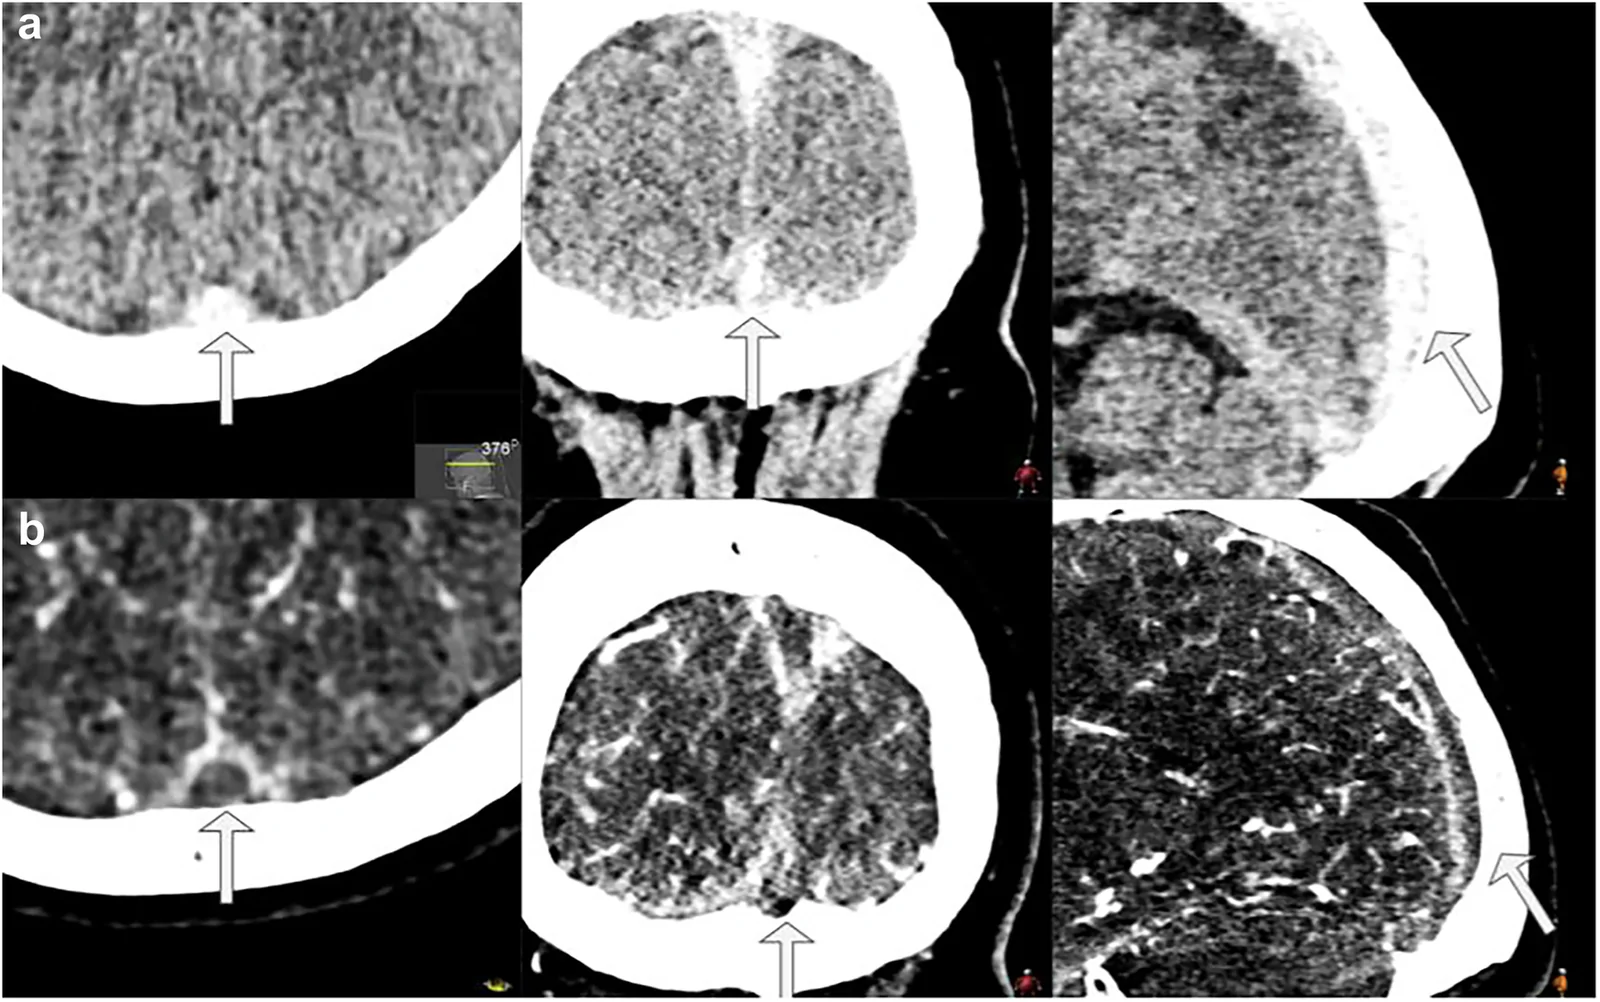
Acute superior sagittal sinus (SSS) thrombosis. a Non-enhanced CT shows hyperattenuation within the SSS (arrow), indicating acute thrombus. b CT venography demonstrates a filling defect in the SSS (arrow) with the absence of contrast opacification, confirming venous occlusion and resembling an “empty delta” sign
